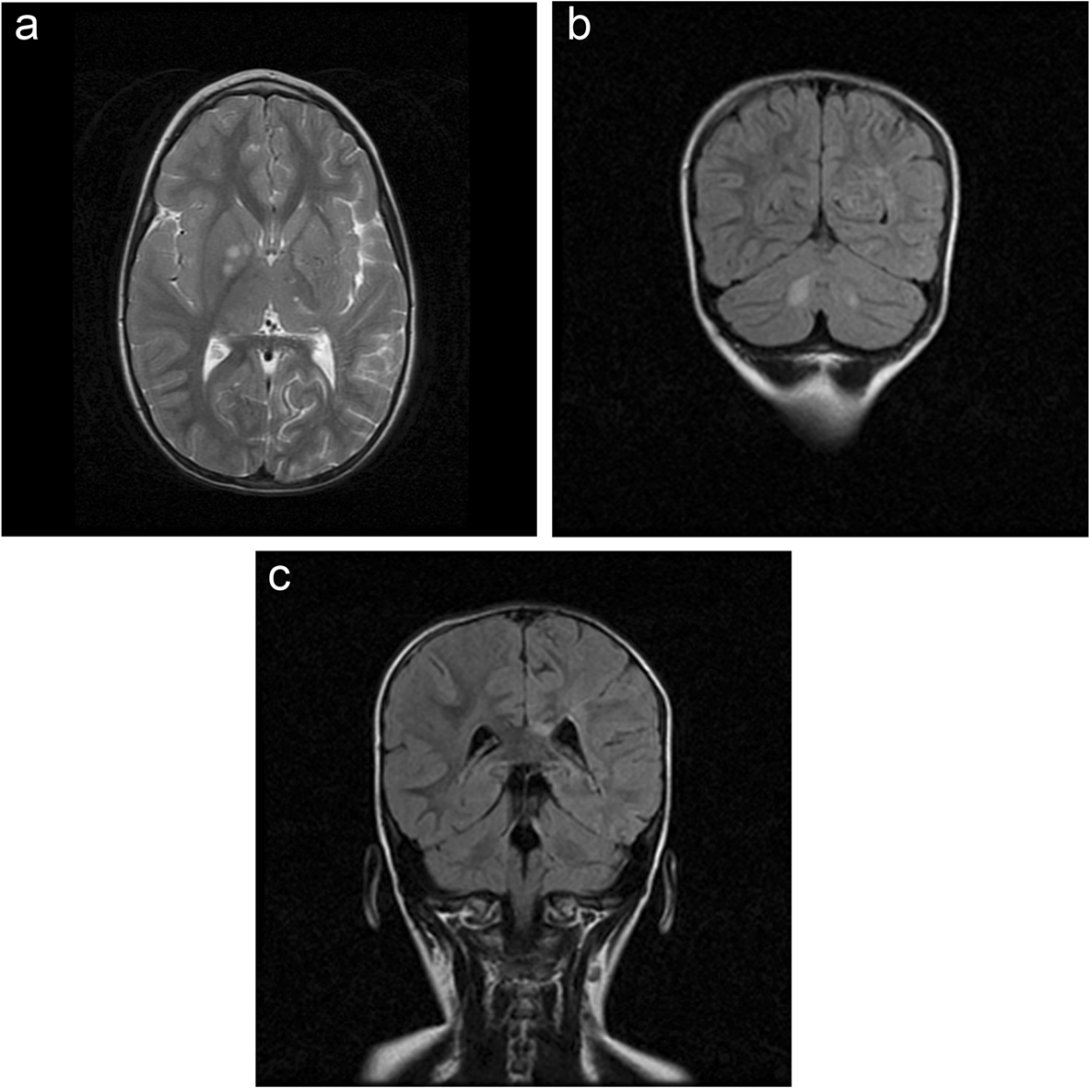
Brain MRI: T2-weighted images (TR/TE 4000/99) showed pathologic areas of increased signal, a. in right basal ganglia (UBO’S), b. Iin the deep white matter of cerebellum, c. in corpus callosum demonstrated typical areas of UBO’S (unidentified bright objects) in NF 1 disease.
